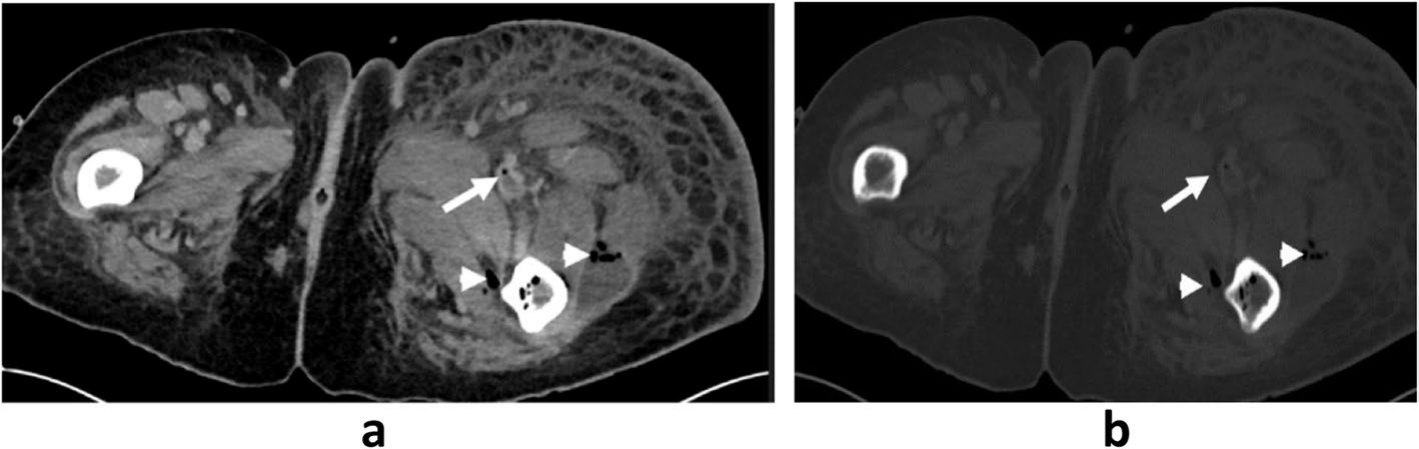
Axial CT section injected in the abdominal and bone window showing thrombosis of the superficial femoral artery seat of air bubble (long arrow) and intramuscular collection seat of air bubble (short arrow)
